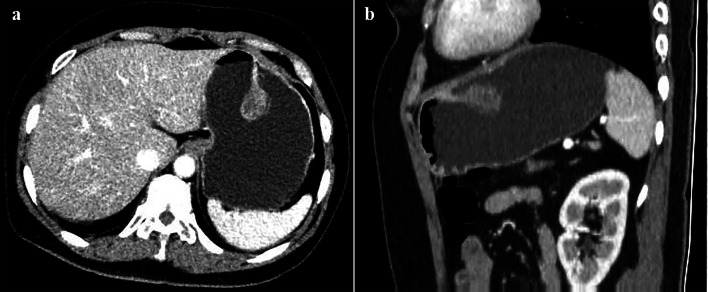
55-year-old woman with GCP revealing a 2.7-cm spherical mass with pedicle. Axial venous phase (a) and sagittal venous phase (b) CT images show a heterogeneous enhanced mass in the greater curvature of gastric body, which is connected to a 2.35-cm pedicle
